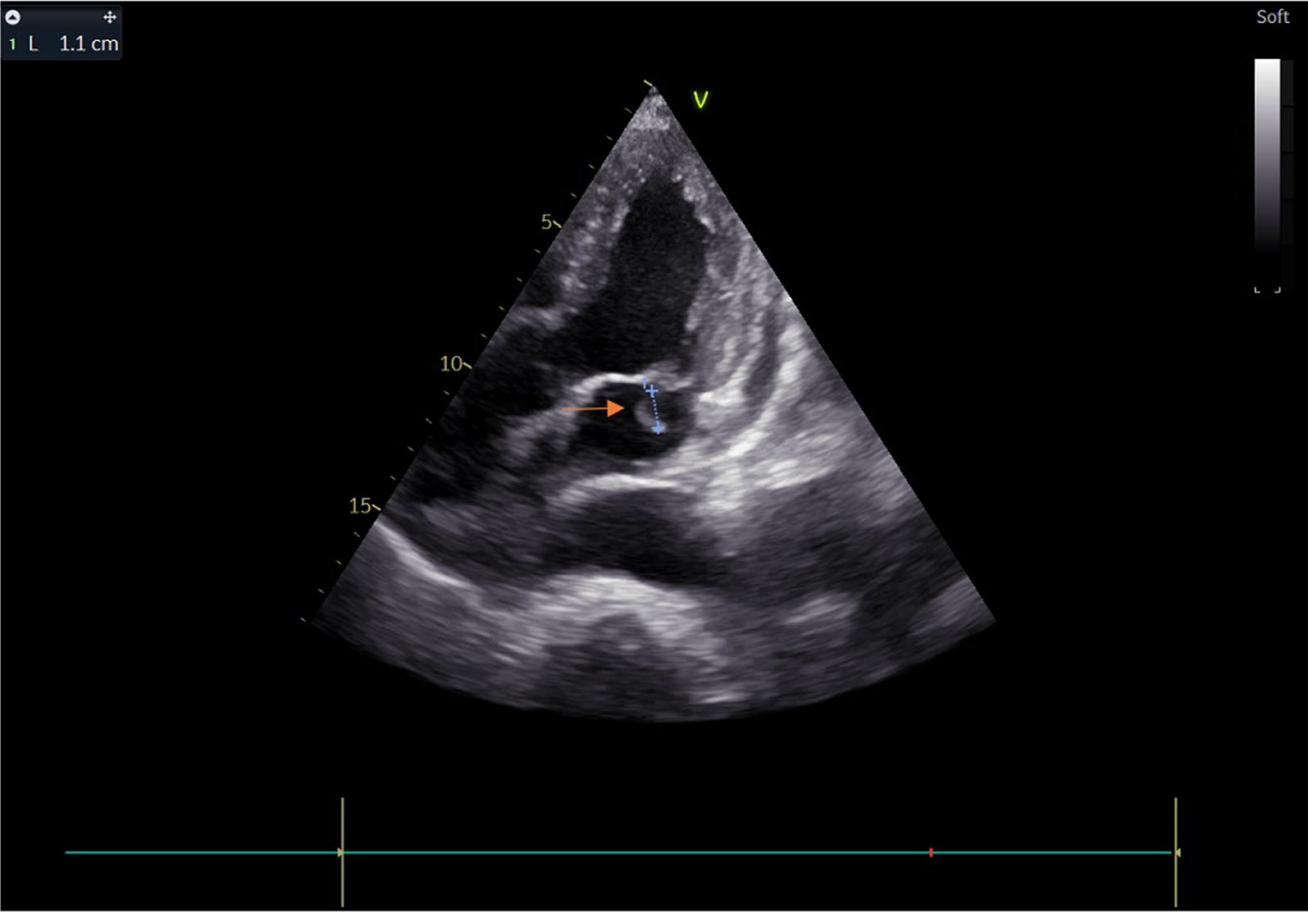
Echocardiographic image showing mitral vegetation on the atrial side
